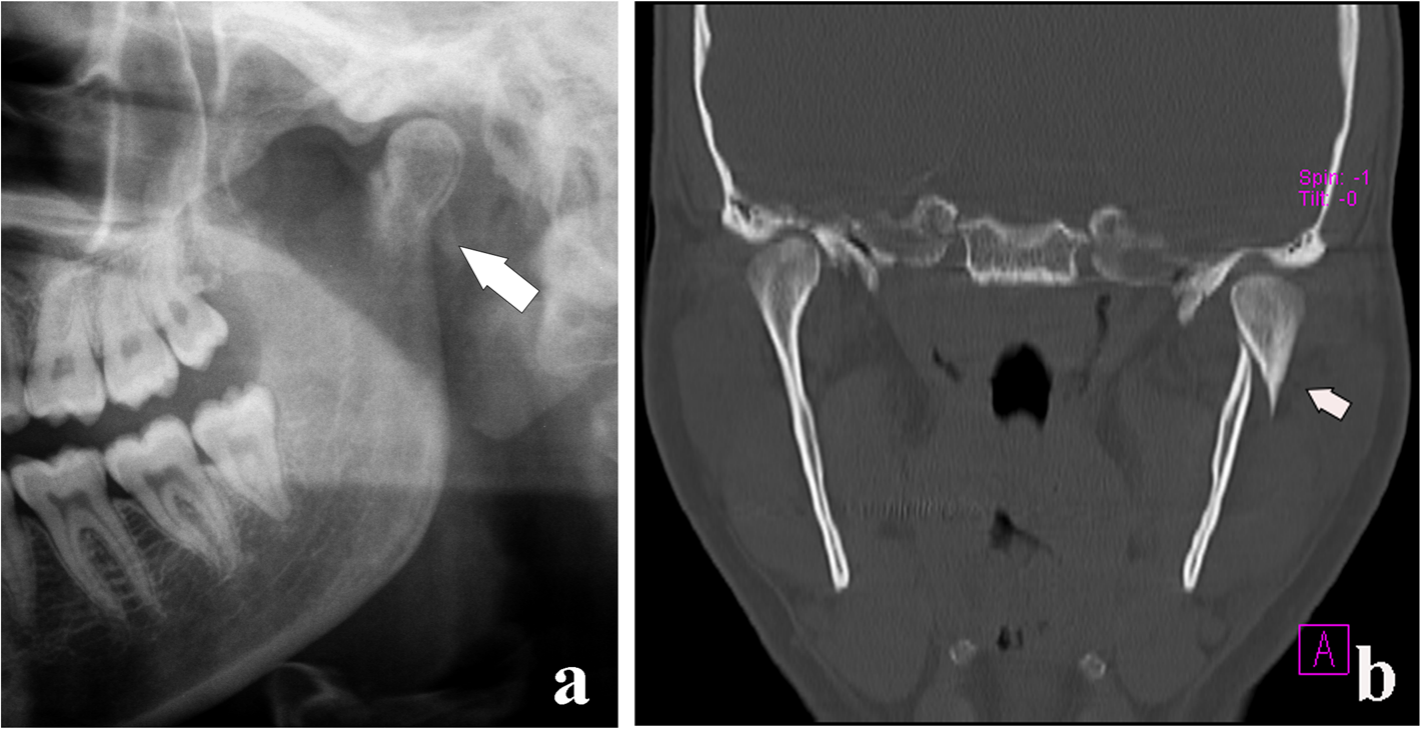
Condylar process fracture. Displacement. a Cropped panoramic radiograph. b Computed tomography coronal section of the same patient. The bone fragments are not in line (arrows), but the condylar head remains within the glenoid fossa
